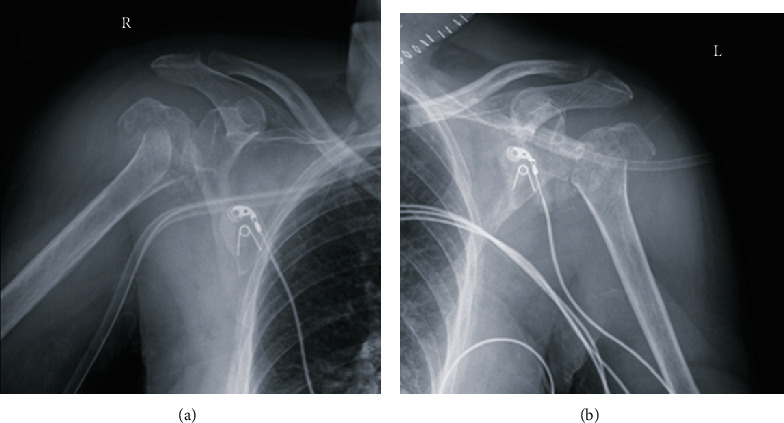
(a) X-ray radiograph of the right shoulder showing a comminuted proximal humerus fracture. (b) X-ray radiograph of the left shoulder showing a comminuted proximal humerus fracture.
